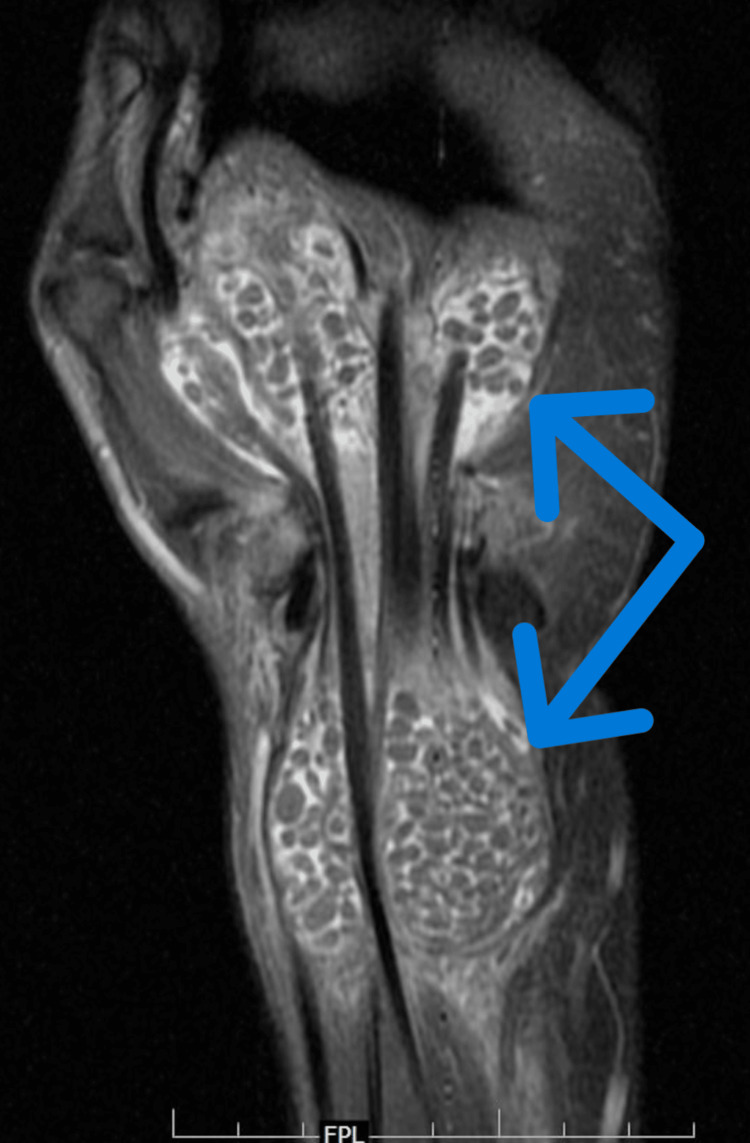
Coronal PDFS MR image showing the hourglass-shaped collection across the carpal tunnel extending from the distal forearm to the level of metacarpal shafts with numerous rice bodies PDFS: proton density fat-saturated
